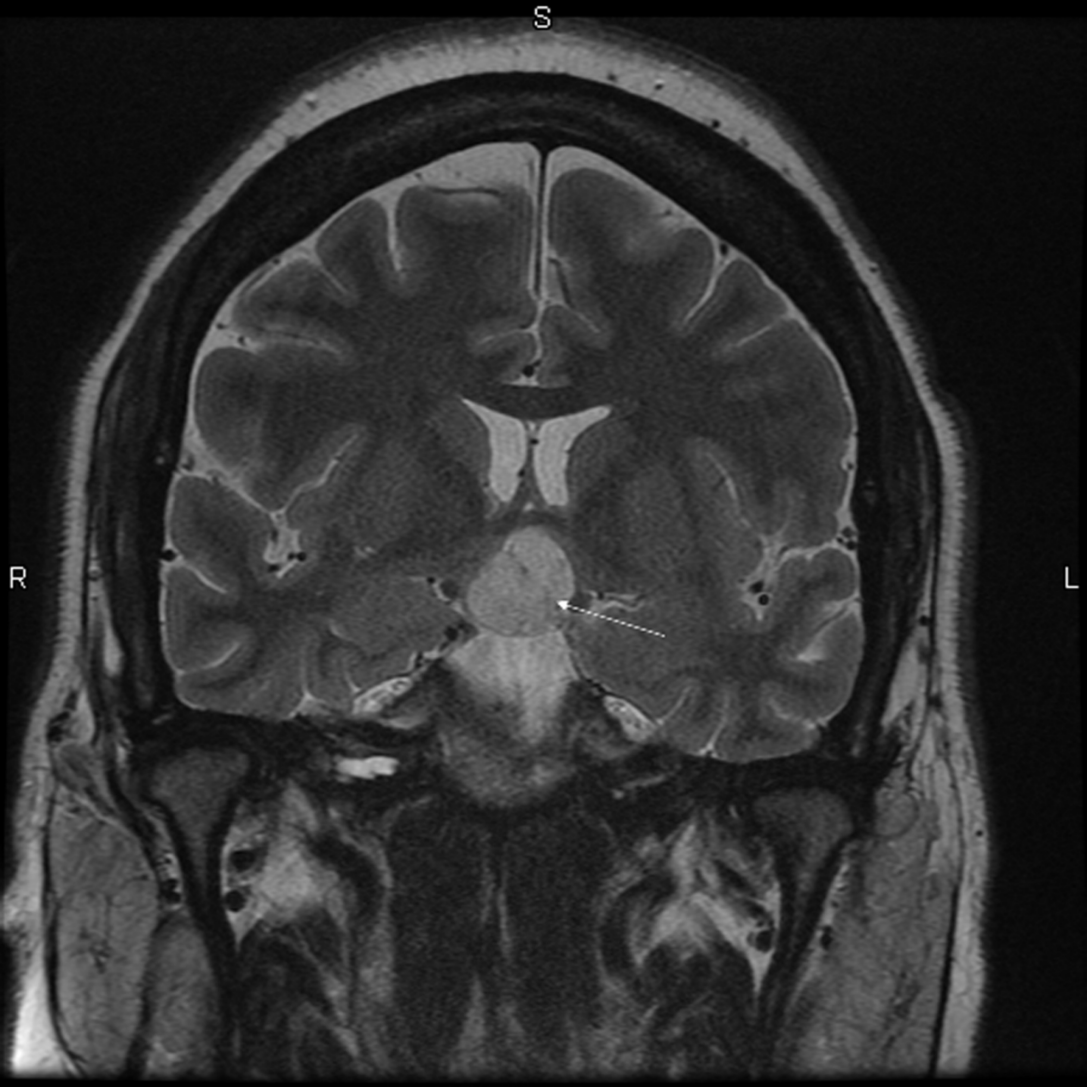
Coronal T2 Preoperative brain MRI showing a multi-locular suprasellar cyst (solid white arrow) measuring approximately 1.6 x 1.8 x 2.4 cm
